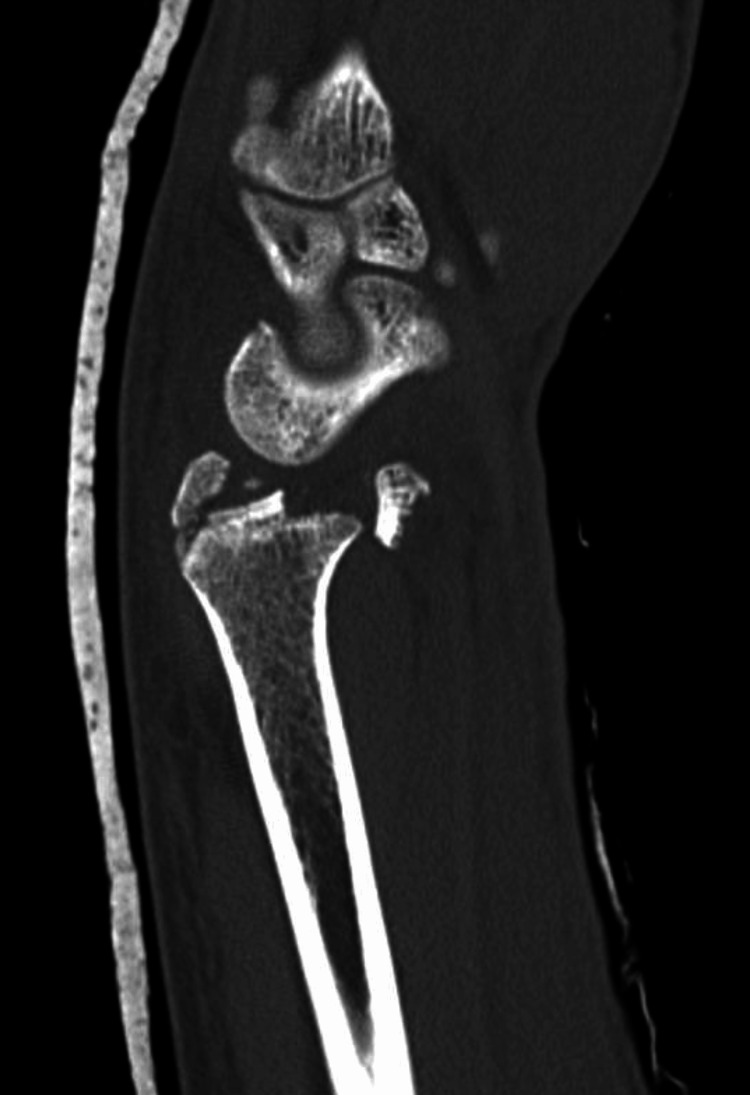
Case example of patient with volar rim fragment that may not be amenable to standard volar plate fixation in isolation. Sagittal CT reconstruction showing complete extrusion of articular fragment of scaphoid fossa in a volar direction.
